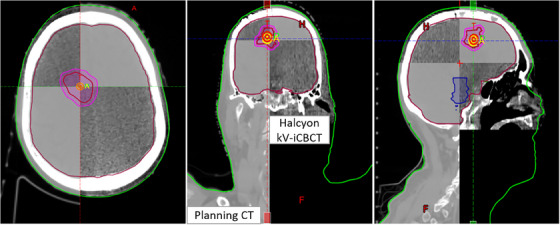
Axial, coronal, and sagittal views of the Halcyon kV‐iCBCT images (see split window in all 3 views) co‐registered with planning CT images used for image guided‐SRT on Halcyon. On top of anatomical landmarks, the GTV (red) and PTV (pink) are shown. The Halcyon kV‐iCBCT images were acquired in the treatment position, 3D rigid‐registration was performed via auto‐matching of online kV‐iCBCT with the planning CT followed by manual refinement of the registration. Planning isocenter (P) and CBCT acquisition isocenter (A) are shown. The setup errors in the first treatment were <2 mm in each direction.
